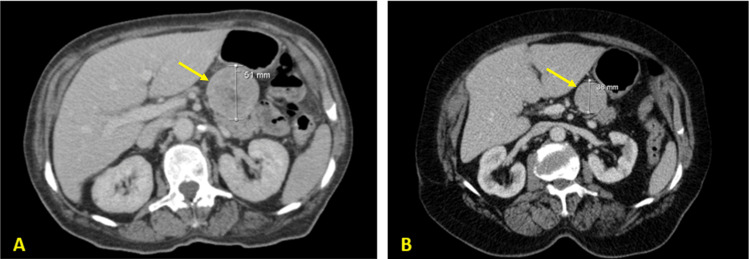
(A) Abdominal computed tomography (CT) scan at diagnosis (maximum diameter: 51 mm). (B) Abdominal CT scan after six months of octreotide long-acting release 20 mg 4/four weeks (maximum diameter: 38 mm).
